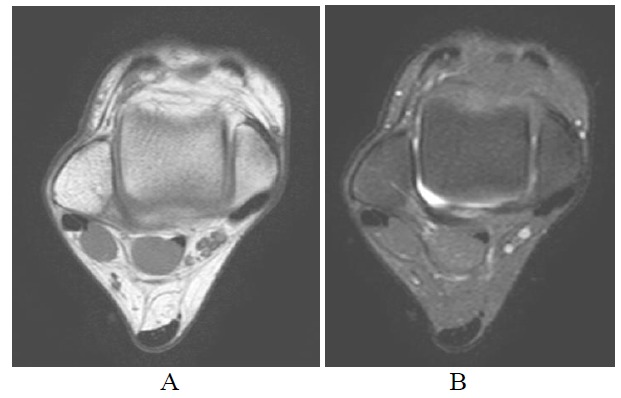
Normal Achilles tendon. T1-weighted and fat suppressed T2-weighted axial images show normal tendon with well defined margin. Its AP dimension should be less than 8mm with concave anterior contour.
